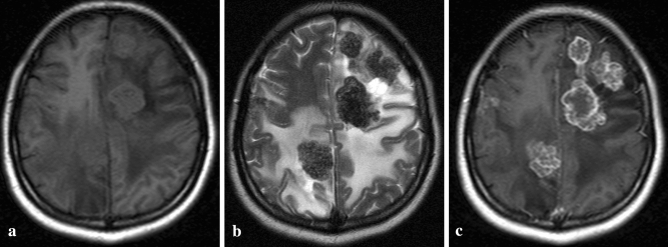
Multiple cerebral lesions were isointense in the T1-weighted image (a) and heterogeneously hypointense in the T2-weighted image (b) with surrounding perilesional edema. All lesions were enhanced peripherally following injection of contrast (c).
